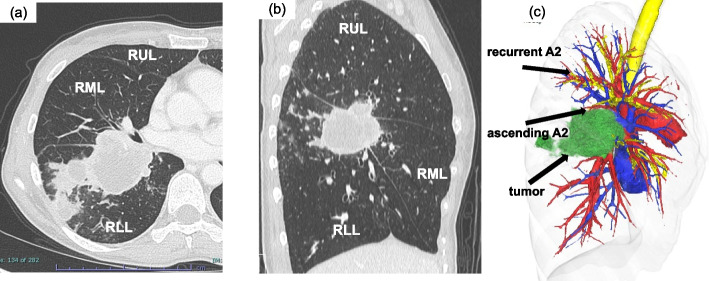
Chest computed tomography (CT) and preoperative three-dimensional CT three weeks after the initial scan (a) Follow-up chest CT obtained three weeks after the initial scan demonstrates rapid enlargement of the tumor. b Sagittal reconstructions show tumor invasion in the middle and upper lobes. c The tumor has invaded to the branching of the ascending pulmonary artery. A recurrent A2 branched from the upper lobe trunk of the pulmonary artery
